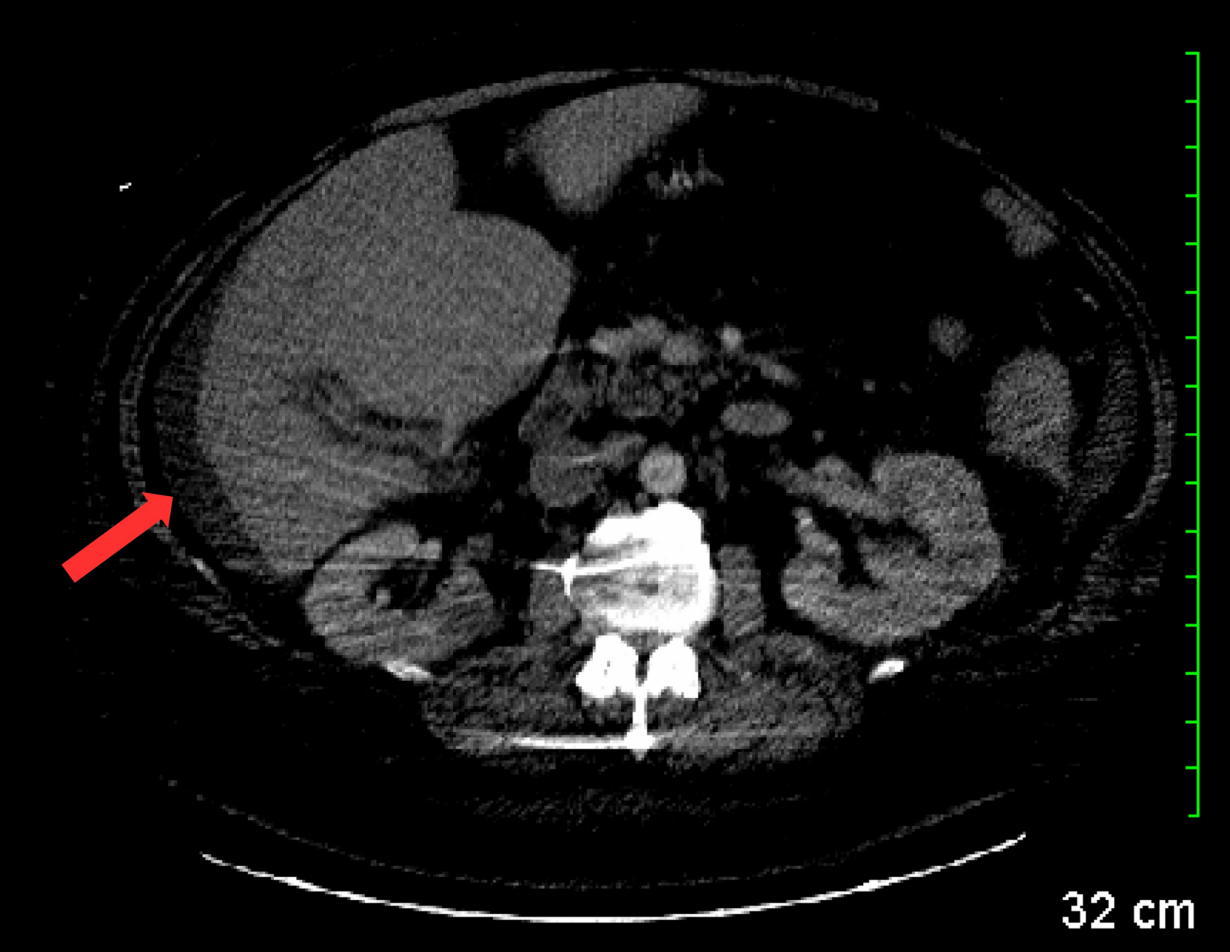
CT scan demonstrating the presence of ascites (red arrow). CT: computed tomography
